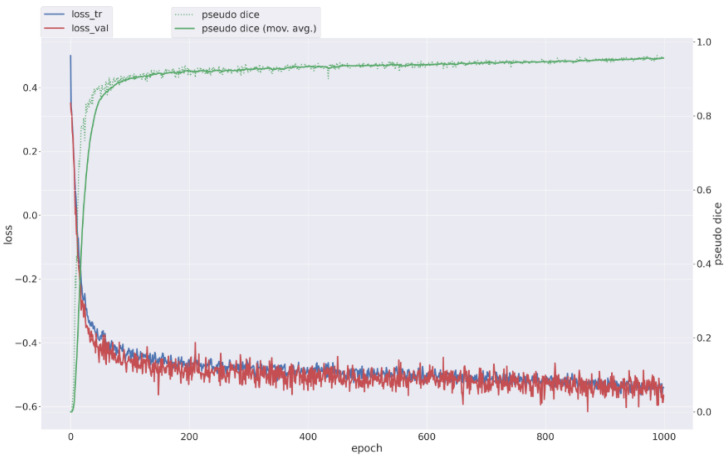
nnU-Nettraining performance on CT. Training and validation loss trajectories with corresponding EMA pseudo-Dice values across epochs, showing stable convergence of the nnU-Net 3d_fullres configuration.
